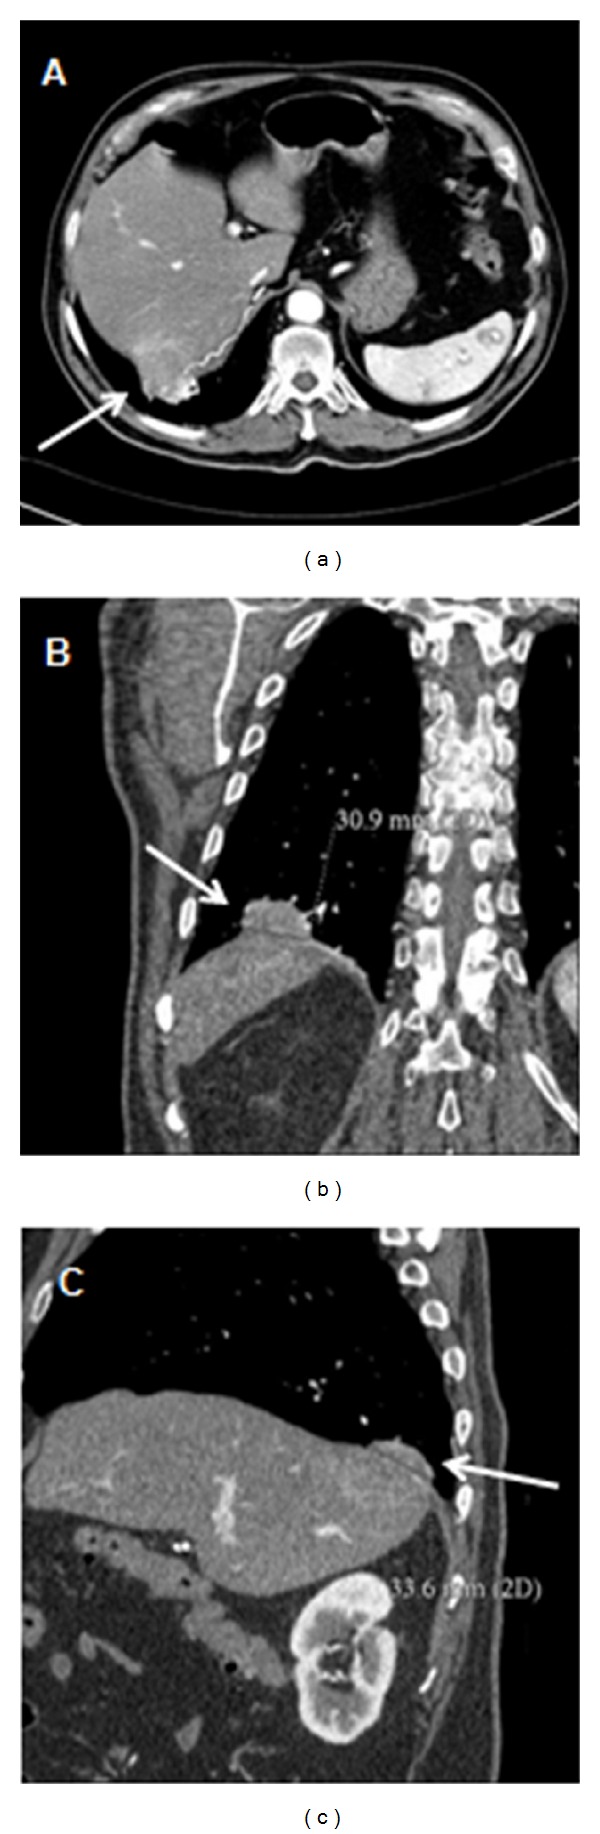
Axial (a), coronal (b), and sagittal (c) images from a computed tomography of neck, thorax, and abdomen with intravenous contrast demonstrating a nodular image close to the diaphragm, in the right side, with transdiaphragmatic infiltration, hepatic invasion, and arterial vascularization (*arrows*).
